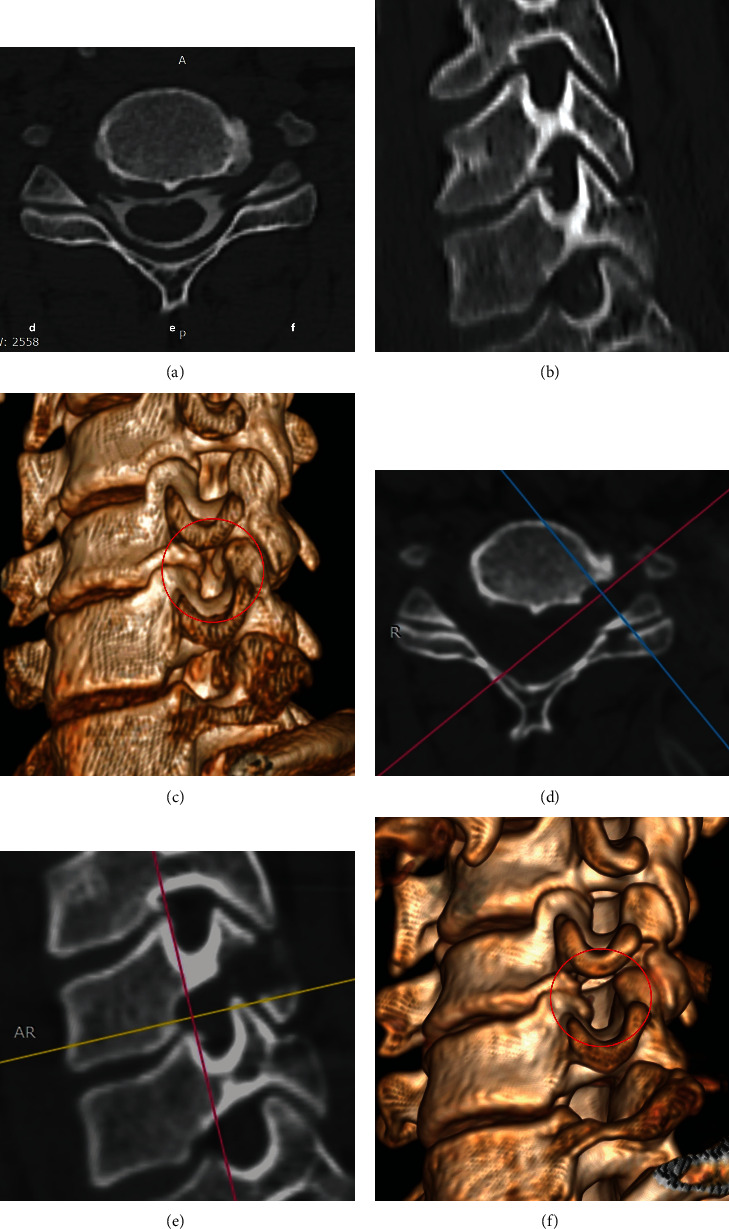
Comparison of CT images before and after decompression from the superior of the exiting root at the C5-6 level. Preoperative images: (a) axial view, (b) sagittal view, and (c) reconstruction image. Postoperative images: (d) axial view, (e) sagittal view, and (f) reconstruction image.
